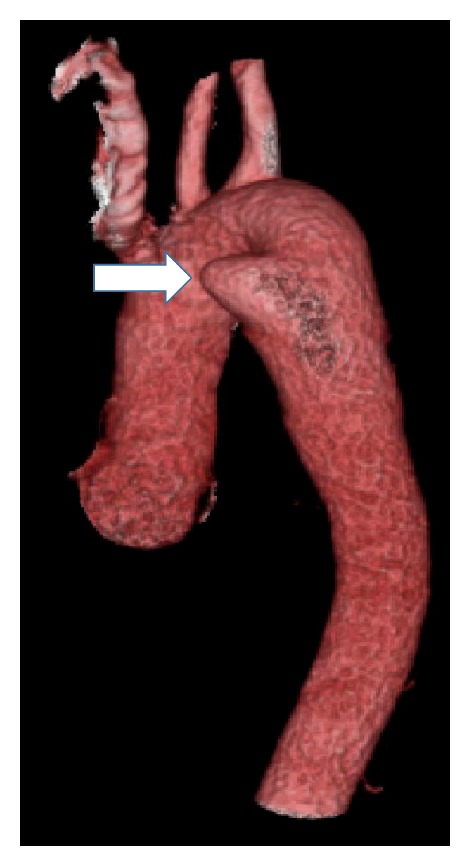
High resolution CT angiogram assisted 3-dimensional reconstruction of the arch of aorta showing the ductus diverticulum (white arrow).
